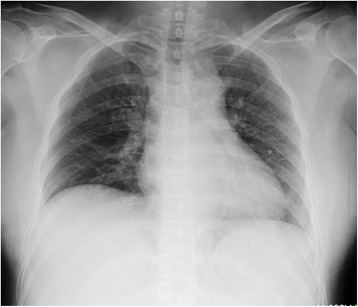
No coins were detectable on the initial anteroposterior chest radiograph
